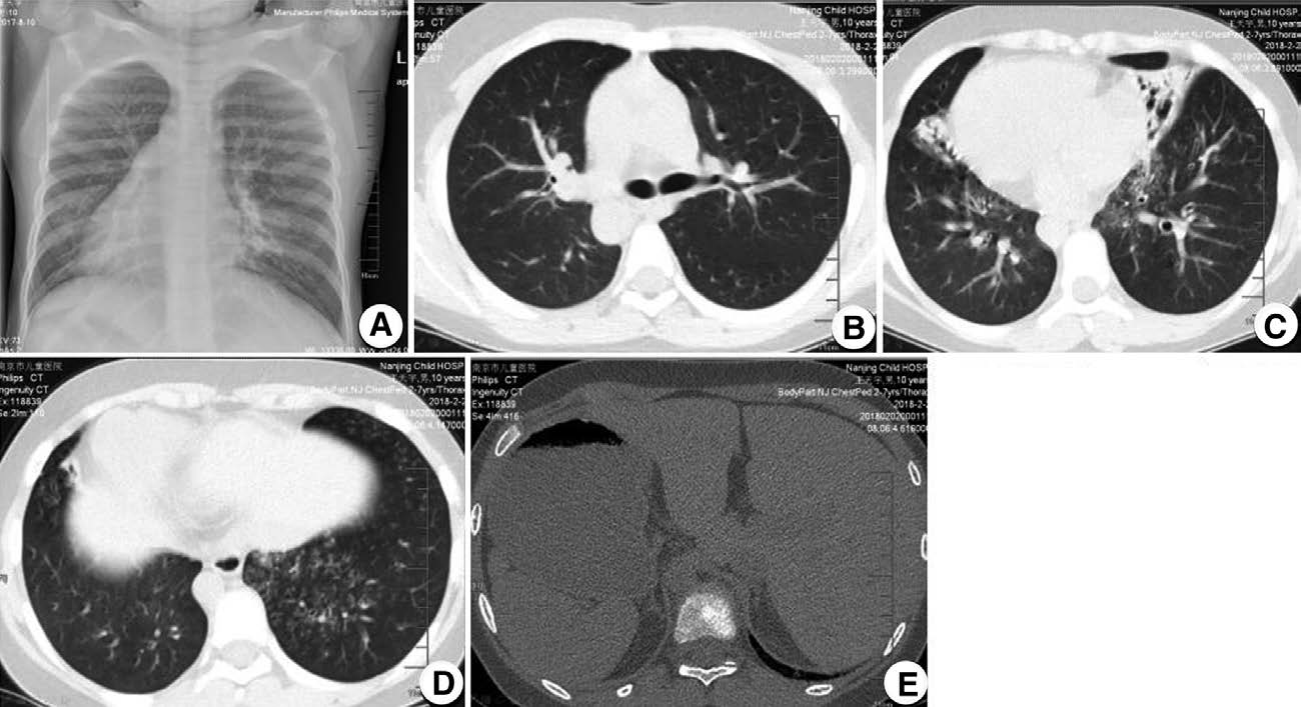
B-mode ultrasound and CT scan showing transposition of the heart, bronchus, stomach, liver, and spleen (A, B, and E); The volume of the right ligule and the left middle lung was shrank, and bronchiectasis was seen. Multiple small patches were noted in the lower lobes.
